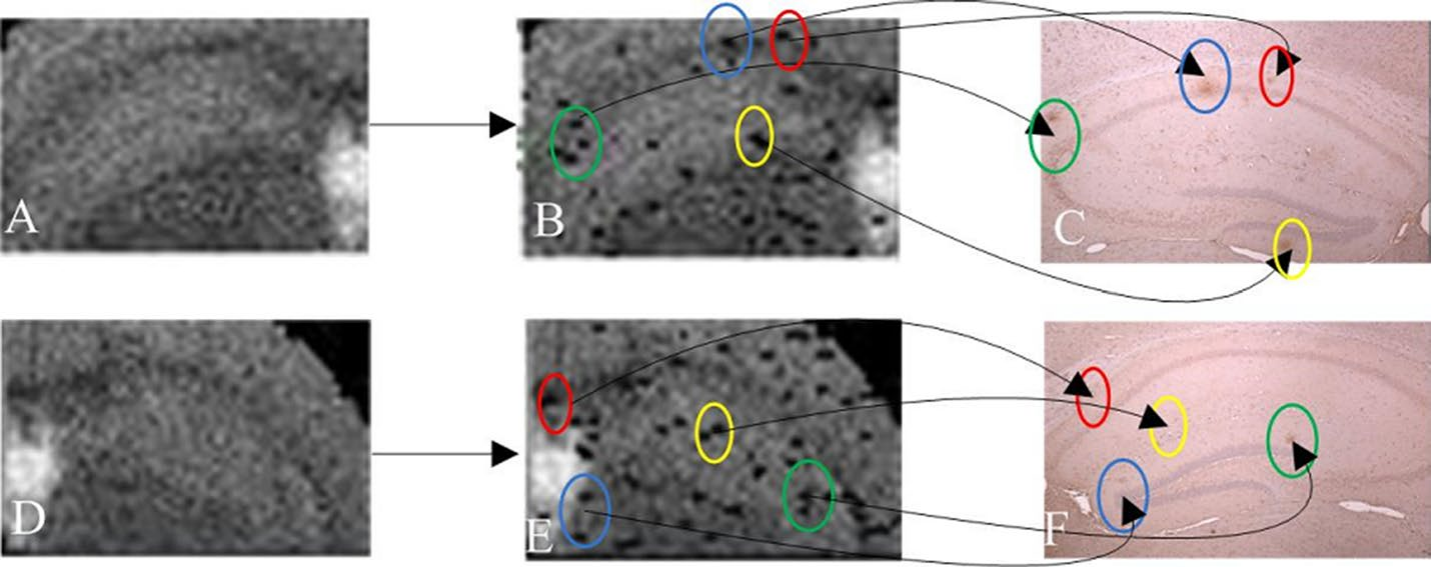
Effect of detection of the Aβ protein deposition. A, D Hippocampi on brain MR images; B, E Marked hippocampi of MR images; C, F Corresponding tissue section images. The regions marked with *different colors* in B and E are related to the distributions of the Aβ plaque deposition in C and F
